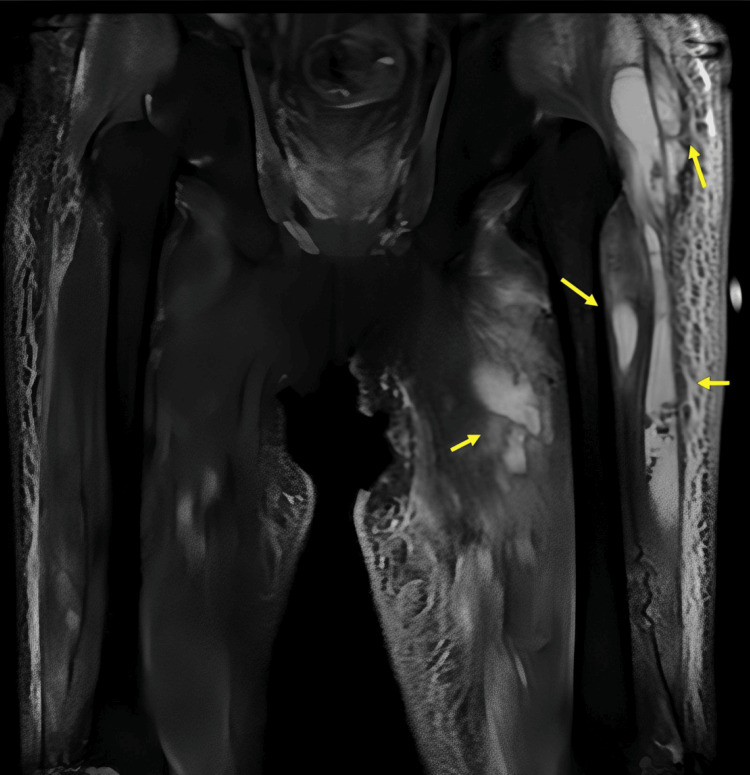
MRI of the thigh On day 21, intramuscular fluid retention with high signal intensity involving the gluteus medius, vastus lateralis, vastus medialis, and adductor brevis muscles is depicted (arrow) on coronal fat-suppressed T2WI. Note that low-signal-intensity spots corresponding to air bubbles are present in the fluid of the vastus lateralis muscle, consistent with an abscess. T2WI, T2-weighted imaging
